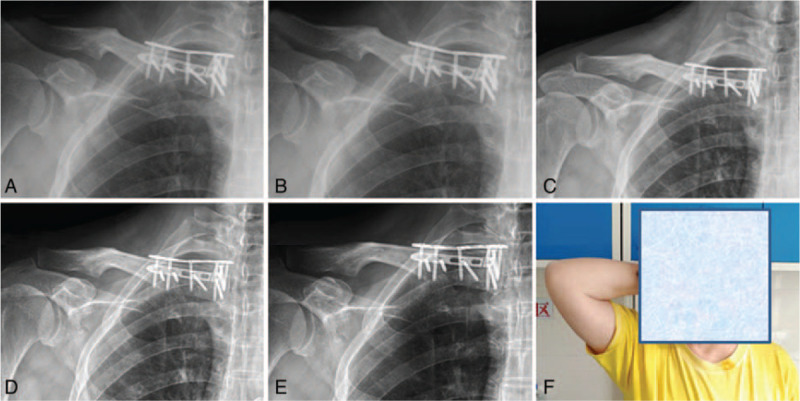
X-ray radiographs at 1 day (A), 3 months (B), 6 months (C), 9 months (D), and 12 months (E) of a female patient after double-plate fixation, as well as the shoulder joint extension (F) at 3 months postoperation.
